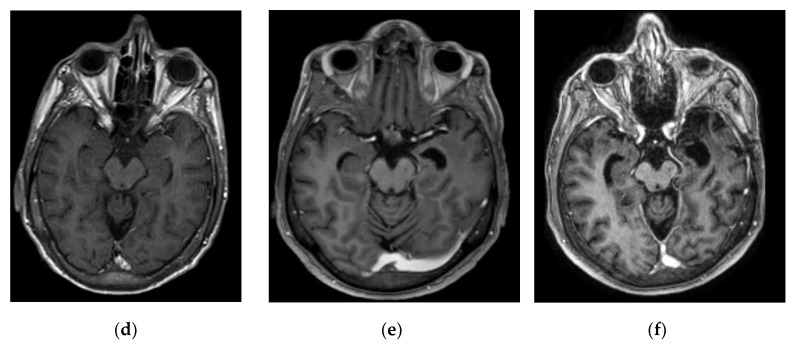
Neuroimaging: (a) FLAIR hyperintensity and swelling in bilateral hippocampi and temporal lobes; (b,c) Progressive worsening of FLAIR hyperintensities with associated atrophy at second and third admissions, respectively; (d) T1 hypointensities in bilateral hippocampi with subtle contrast enhancement; (e,f) Progressive worsening of T1 hypointensities without any contrast enhancement, with associated atrophy at second and third admissions, respectively.
